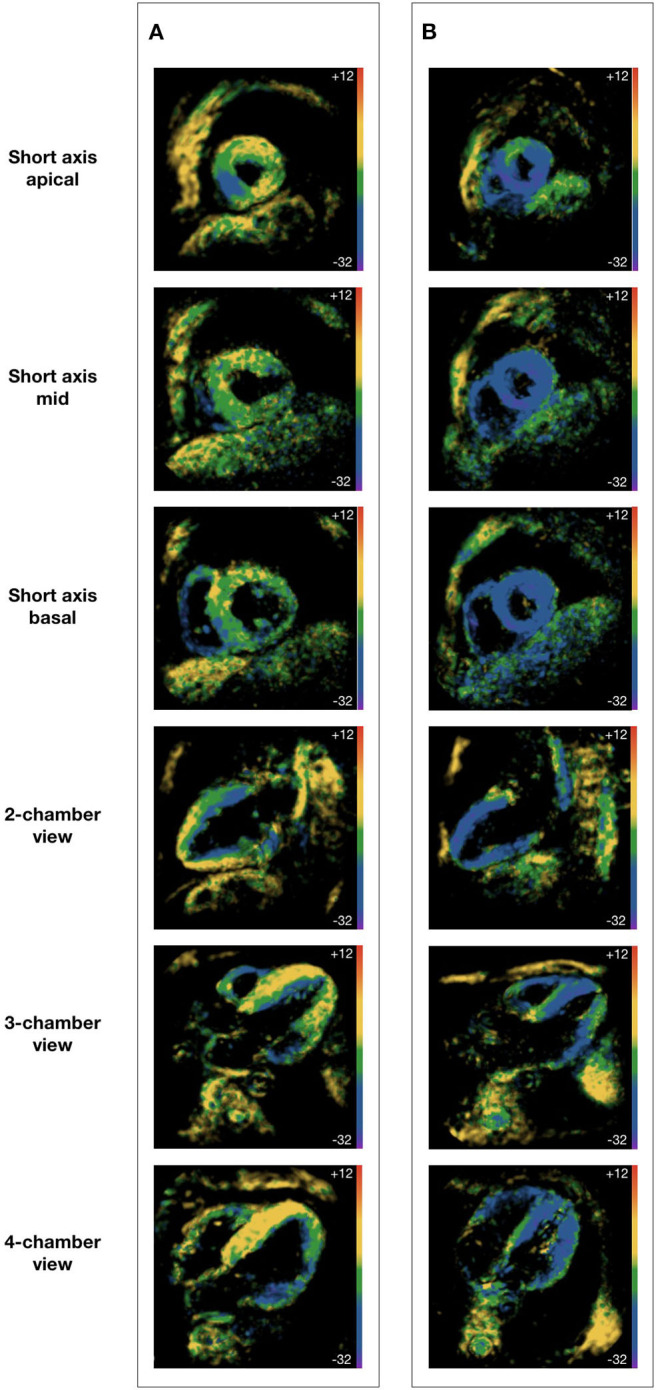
Fast strain-encoded cardiac magnetic resonance imaging. (A) 62 year-old male with heart failure with reduced ejection fraction; (B) 62 year-old male without heart disease; all subjects were at physical rest during image acquisition; all images were acquired at end-systole; global longitudinal strain is derived from short axis views at apical, mid and basal level; global circumferential strain is derived from two-, three-, and for-chamber views.
